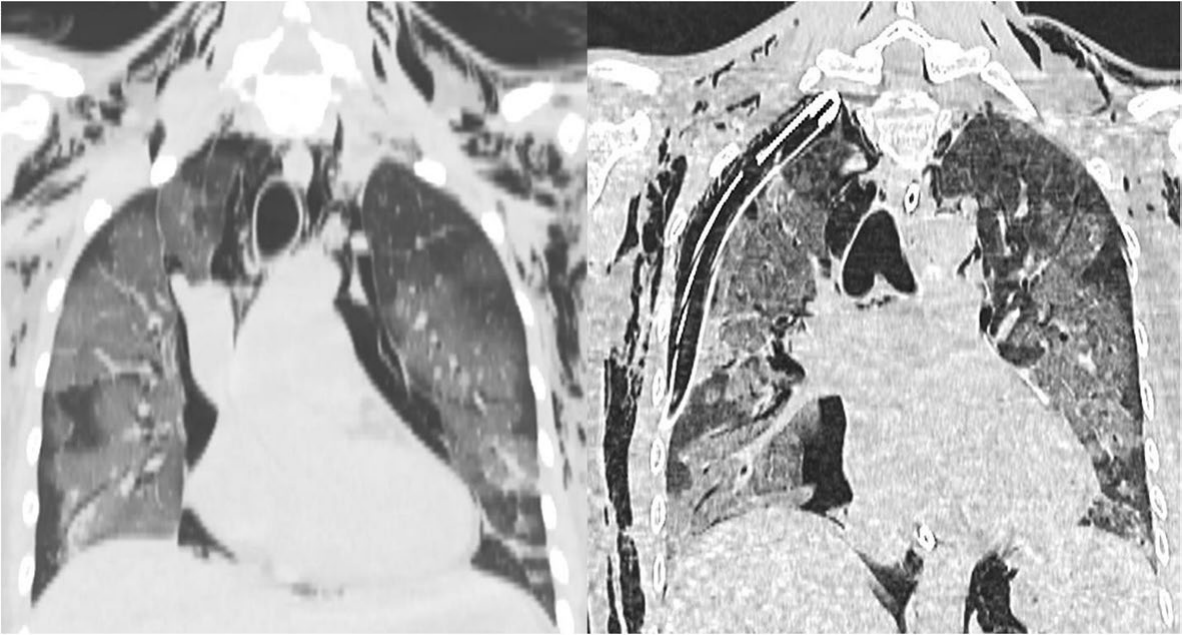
Coronal chest computed tomography images of a 30-year-old female with COVID-19-related bilateral interstitial pneumonia, right pneumothorax, pneumomediastinum, and subcutaneous emphysema before (left) and after (right) chest tube placement
